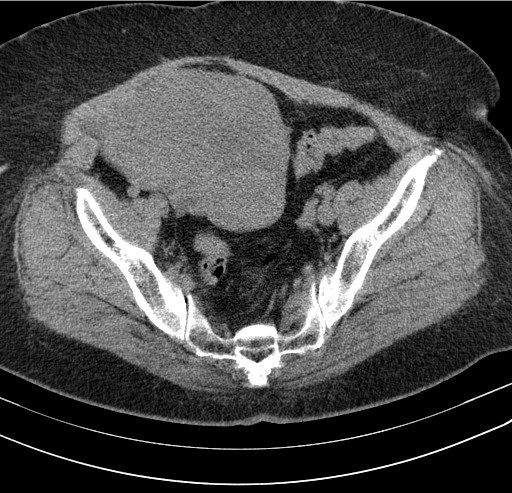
Pelvic computed tomography showing rotated mass toward the contralateral side of the pelviswithout lymphadenopathy or pelvic effusion
